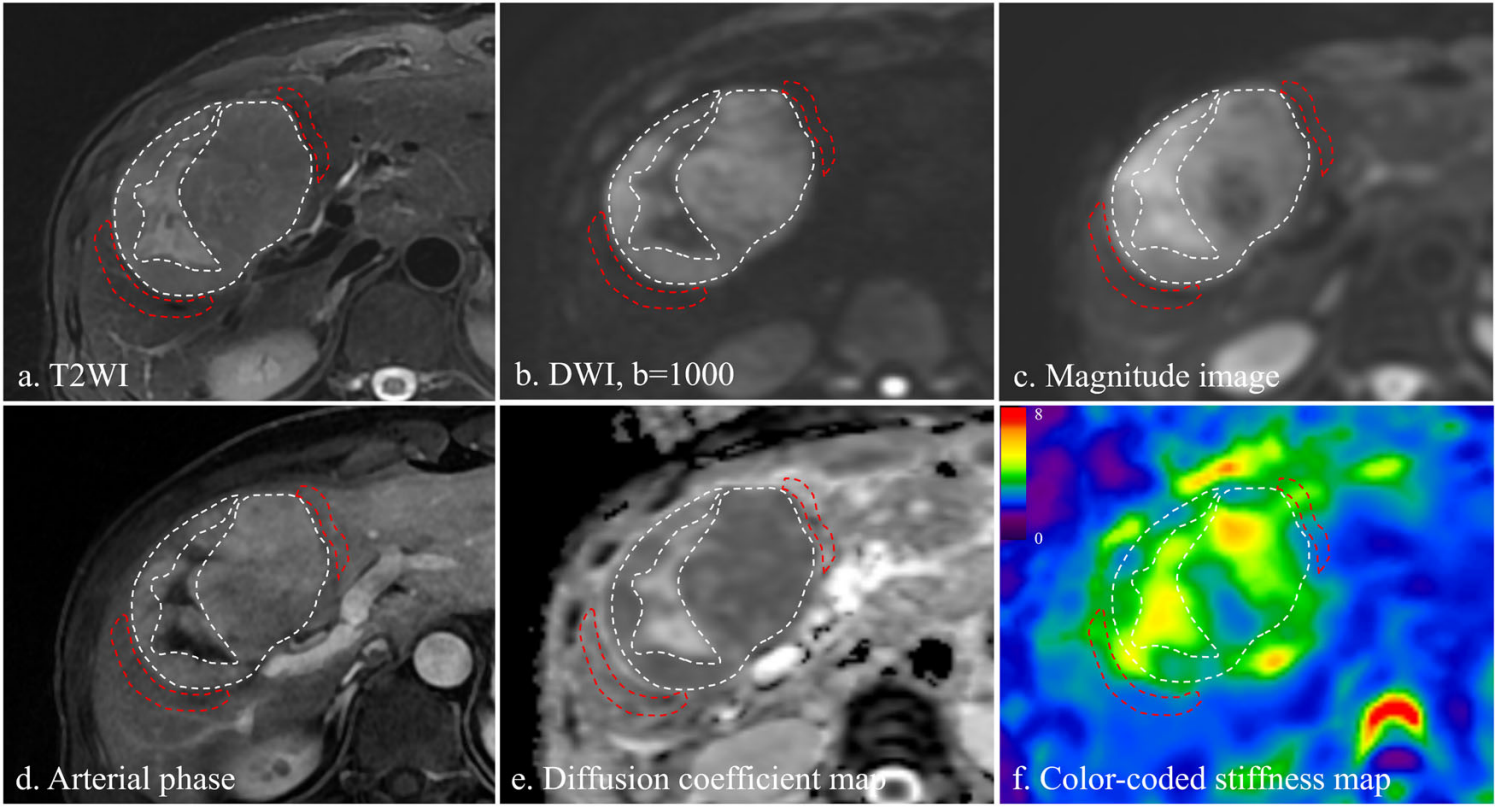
The measurement of apparent diffusion coefficient and stiffness in the tumor (white) and peri-tumor area (red). a T2 weighted image; b diffusion-weighted image; c the T2-weighted magnitude image of magnetic resonance elastography; d late arterial phase of dynamic contrast enhanced imaging; e the apparent diffusion coefficient map; f the color-coded stiffness map
